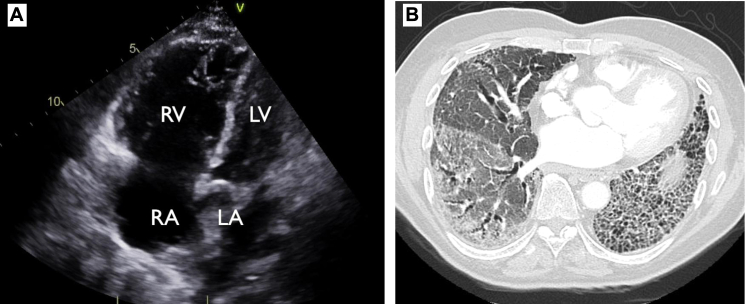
Initial diagnostic testing. Representative images of (A) transthoracic echocardiography apical 4-chamber view demonstrating enlargement of RA and RV, and (B) high-resolution chest CT demonstrating basilar-dependent ground-glass opacities and peripheral subpleural reticulations with honeycombing. LA = left atrium; LV = left ventricle; RA = right atrium; RV = right ventricle.
